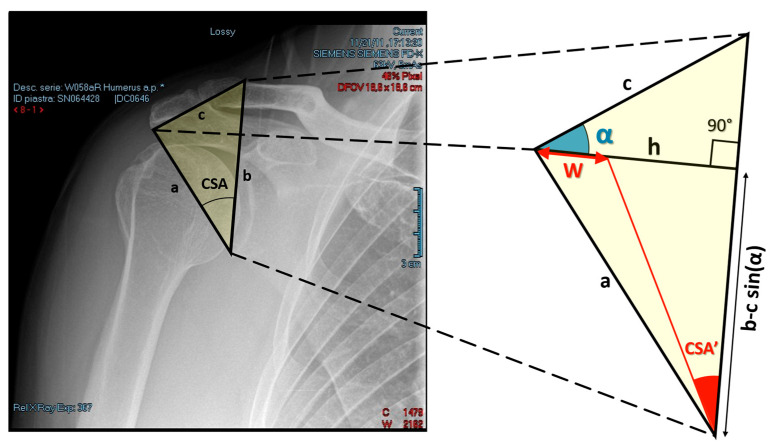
Geometrical derivation of the formula to estimate the amount of lateral resection, w, to obtain a desired postoperative CSA, namely CSA’. Parameters a, b, and c are the radiographic measures needed for the computation. CSA: critical shoulder angle.
